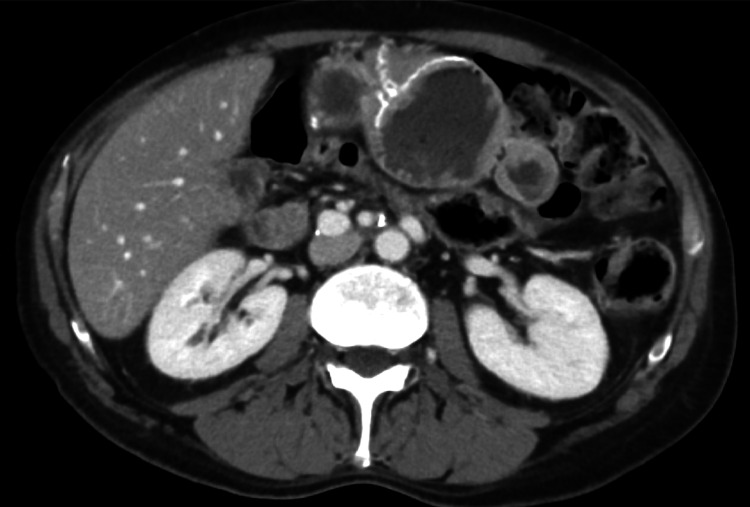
Axial CECT image demonstrating tissue bed post-Whipple procedure There are scar changes without local reoccurrence. CECT: contrast-enhanced computed tomography
